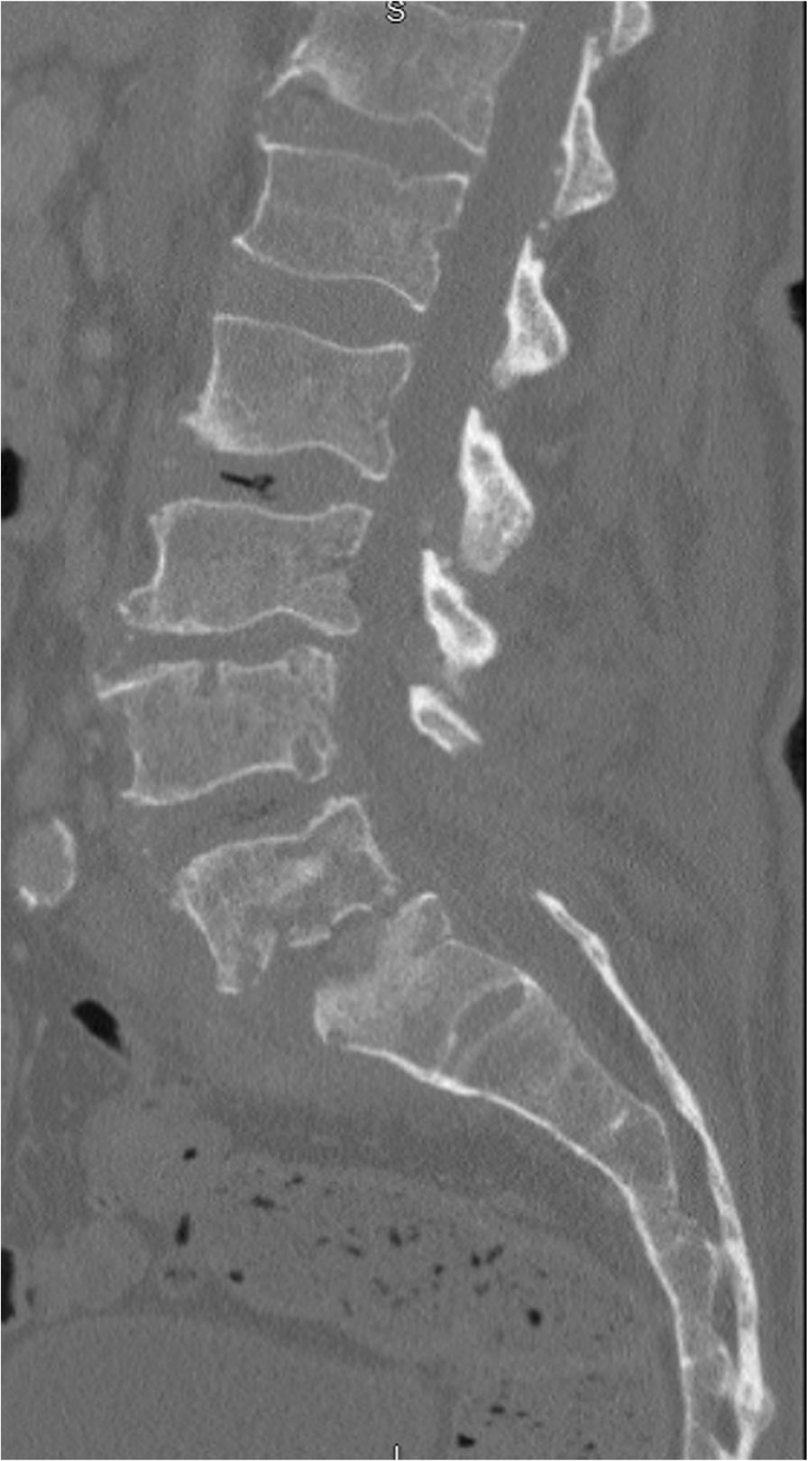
Lumbar CT Sagittal Reconstruction: progression of osteomyelitis at L4 and L5 with progressed anterolisthesis of L5
